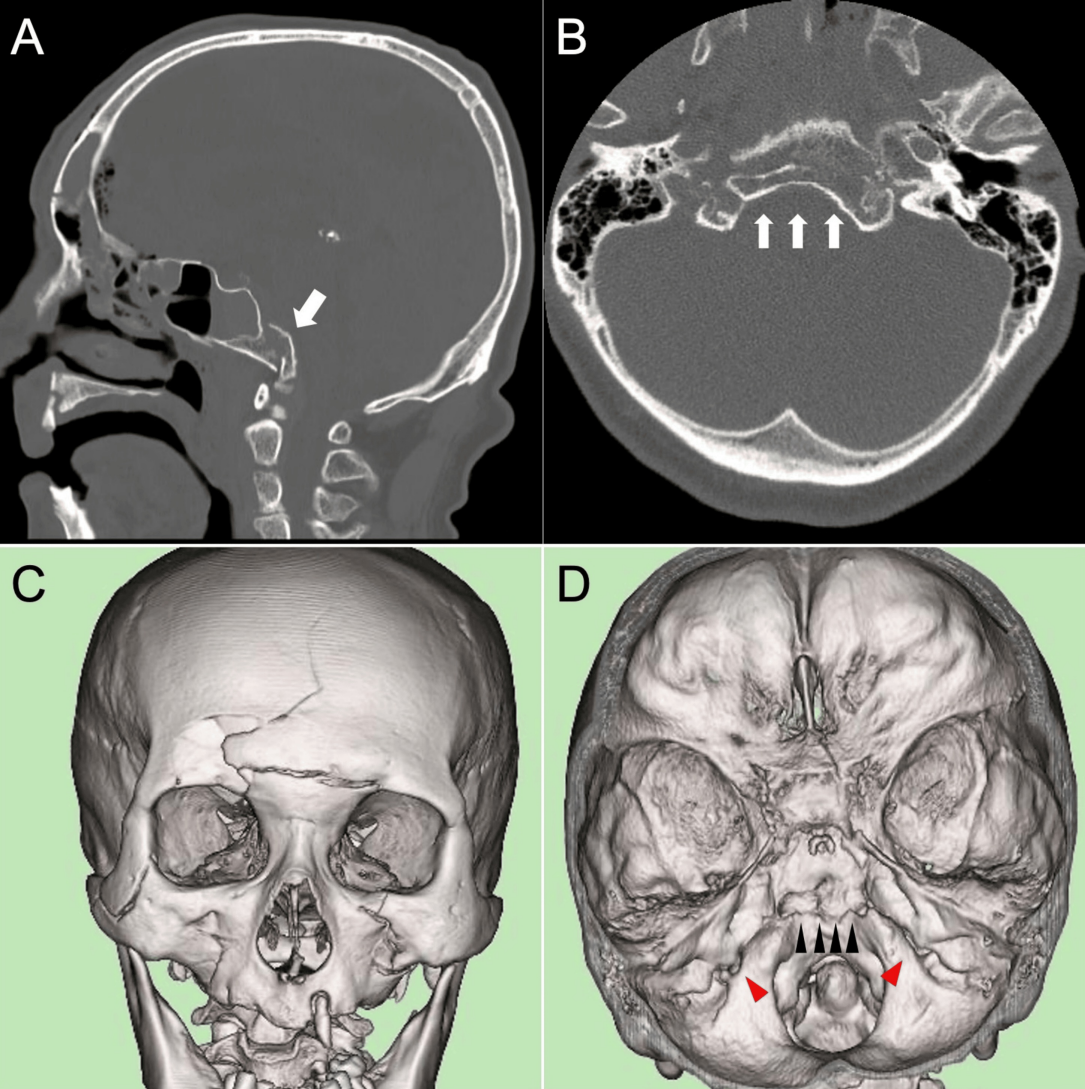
Imaging findings on the initial bone-window computed tomography (CT) in sagittal (A) and axial (B) views and three-dimensional (3D) reconstruction of CT of the face (C) and skull base (D). A: White arrow indicates the clival fracture and the entire head moves downward from its original position. B: The displaced fractured bone fragment is indicated by white arrows. C: The 3D reconstructed facial bone image from the front view shows multiple depressed facial bone fractures, suggesting the impact force was applied directly in front of the face. D: The fracture line is indicated with black arrowheads in the 3D skull base reconstruction from a posterior superior view. Red arrowheads indicate jugular foramina narrowed by the displaced fractured bone fragments.
